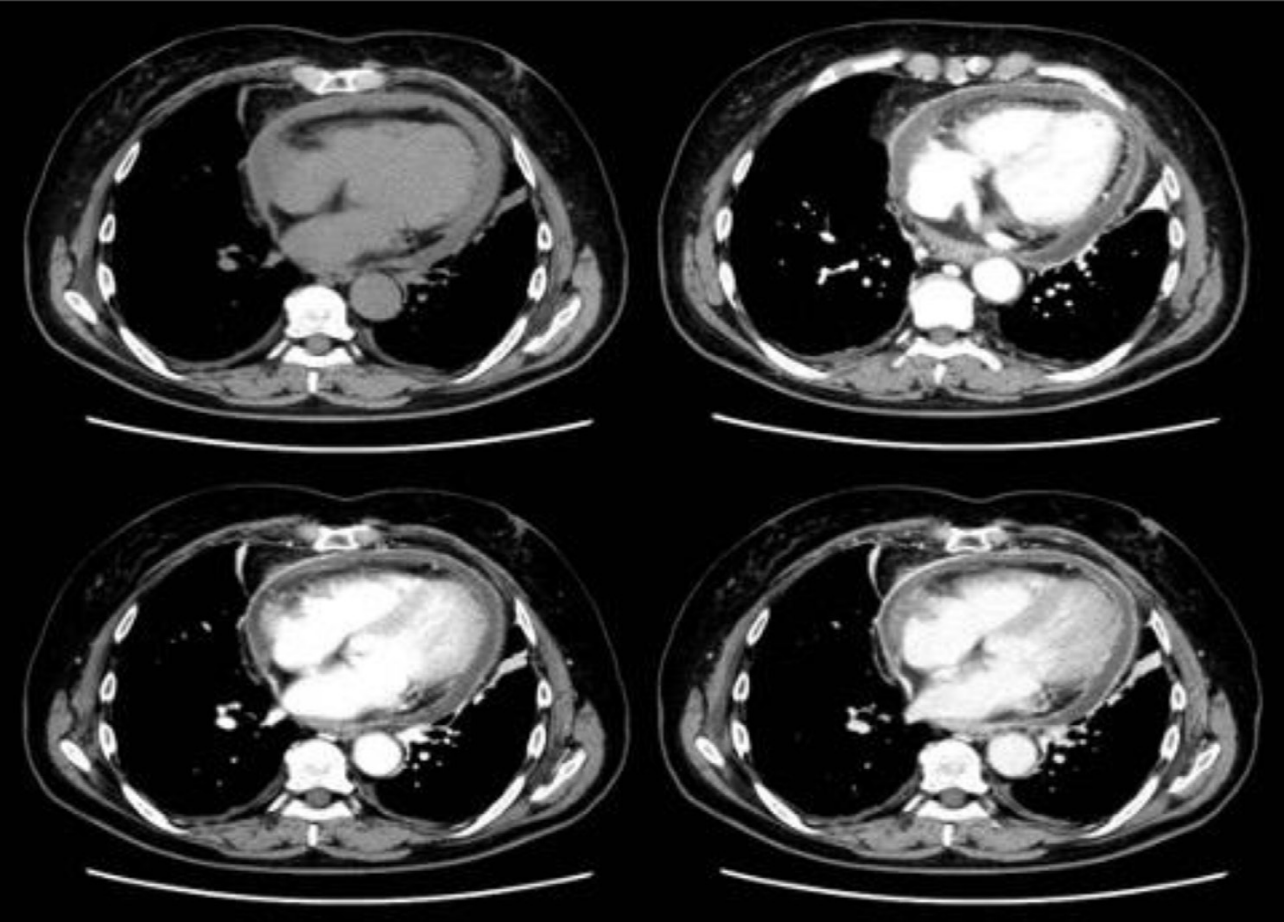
CT images revealed pericardium thickening and enhancement with a pericardial effusion that showed slightly high density compared with water (approximately 20 HU).
